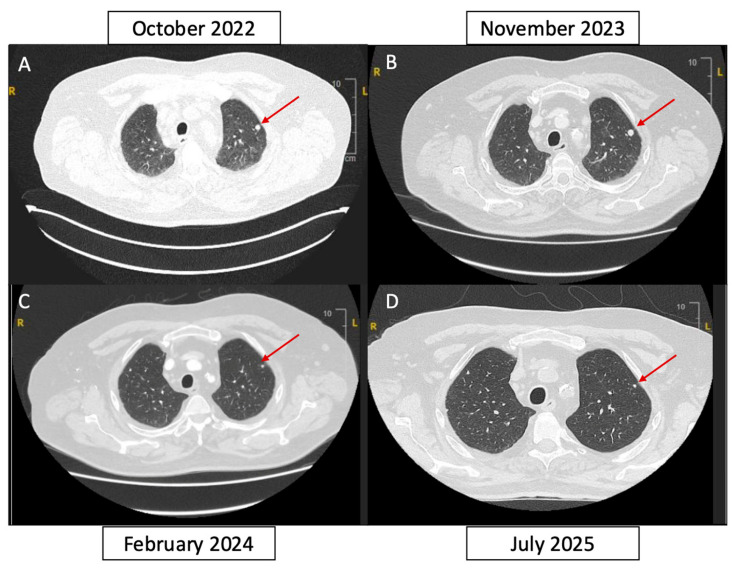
Radiological evolution of pulmonary metastases between 2022 and 2025 in the absence of systemic therapy. (A) Chest CT scan from October 2022 showing a 9 mm subpleural pulmonary nodule. (B) November 2023 CT scan demonstrating slow progression of pulmonary metastases prior to methotrexate discontinuation and cryoablation of the symptomatic bone lesion. (C) February 2024 CT scan revealing spontaneous regression with marked reduction in the size of pulmonary metastases (subpleural nodule: 9 mm → 2 mm). (D) July 2025 CT scan confirming continued tumor regression and a durable partial response according to RECIST 1.1. The red arrow indicates the lung tumor.
